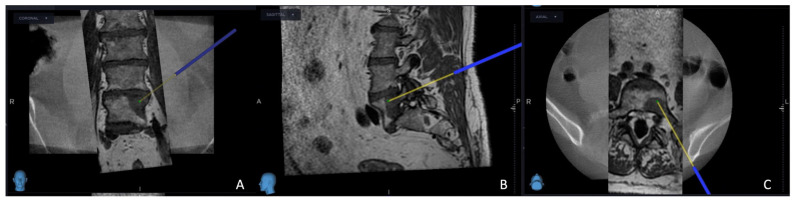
O-arm and MRI fused image, using the MRI image primarily, the yellow projection line, which presents the targeted site for electrode placement: (A) coronal view; (B) sagittal view; (C) axial view.
